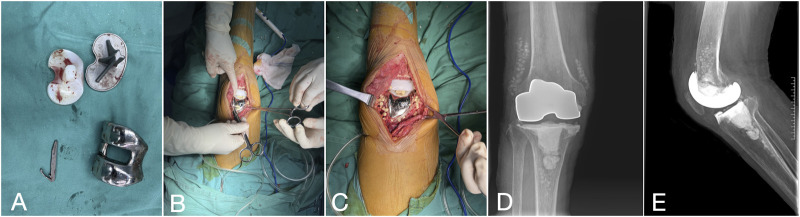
The first-stage procedure. (A) Extraction of the infected prosthesis (B, C) Involved implanting a modified articulating spacer and positioning antibiotic-loaded calcium sulfate pellets around the prosthesis. (D, E) Two weeks post-surgery, the right knee joint’s positive and lateral radiographs display a properly positioned spacer with gradual dissolution of the antibiotic-loaded calcium sulfate beads.
